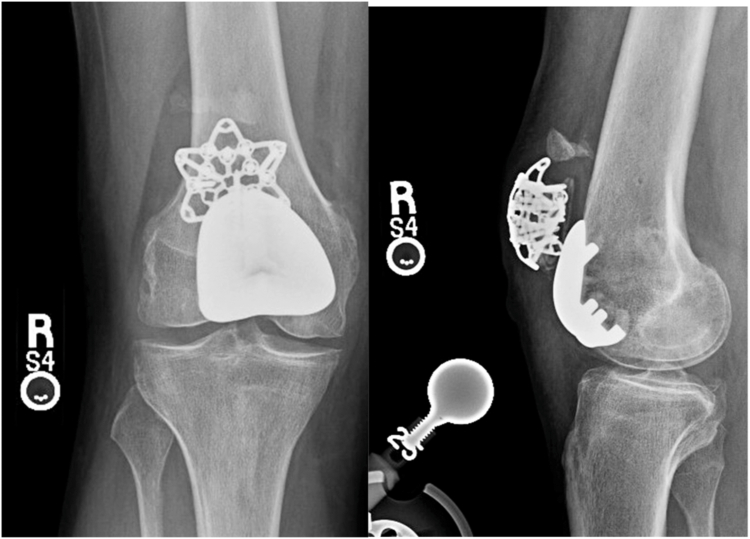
Postoperative anterior-posterior (AP) and lateral radiographs after patella open reduction internal fixation (ORIF) and extensor mechanism repair.
